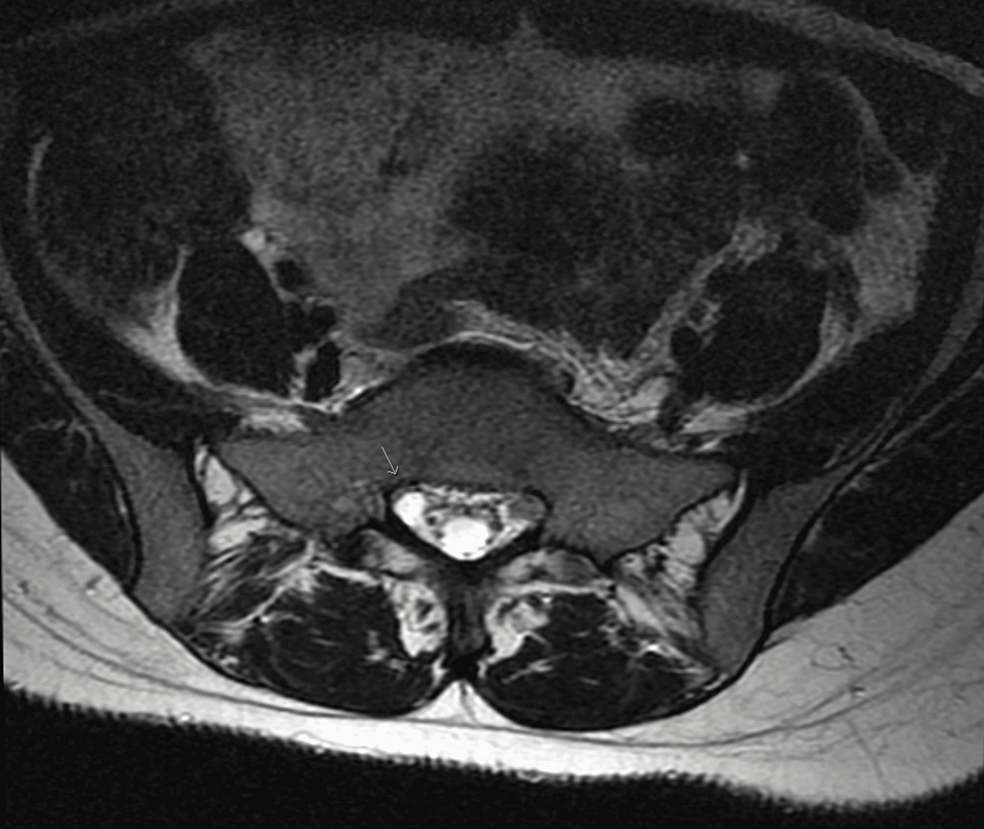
Axial lumbar MRI showing a tiny Tarlov cyst on the right side indicated by an arrow.
